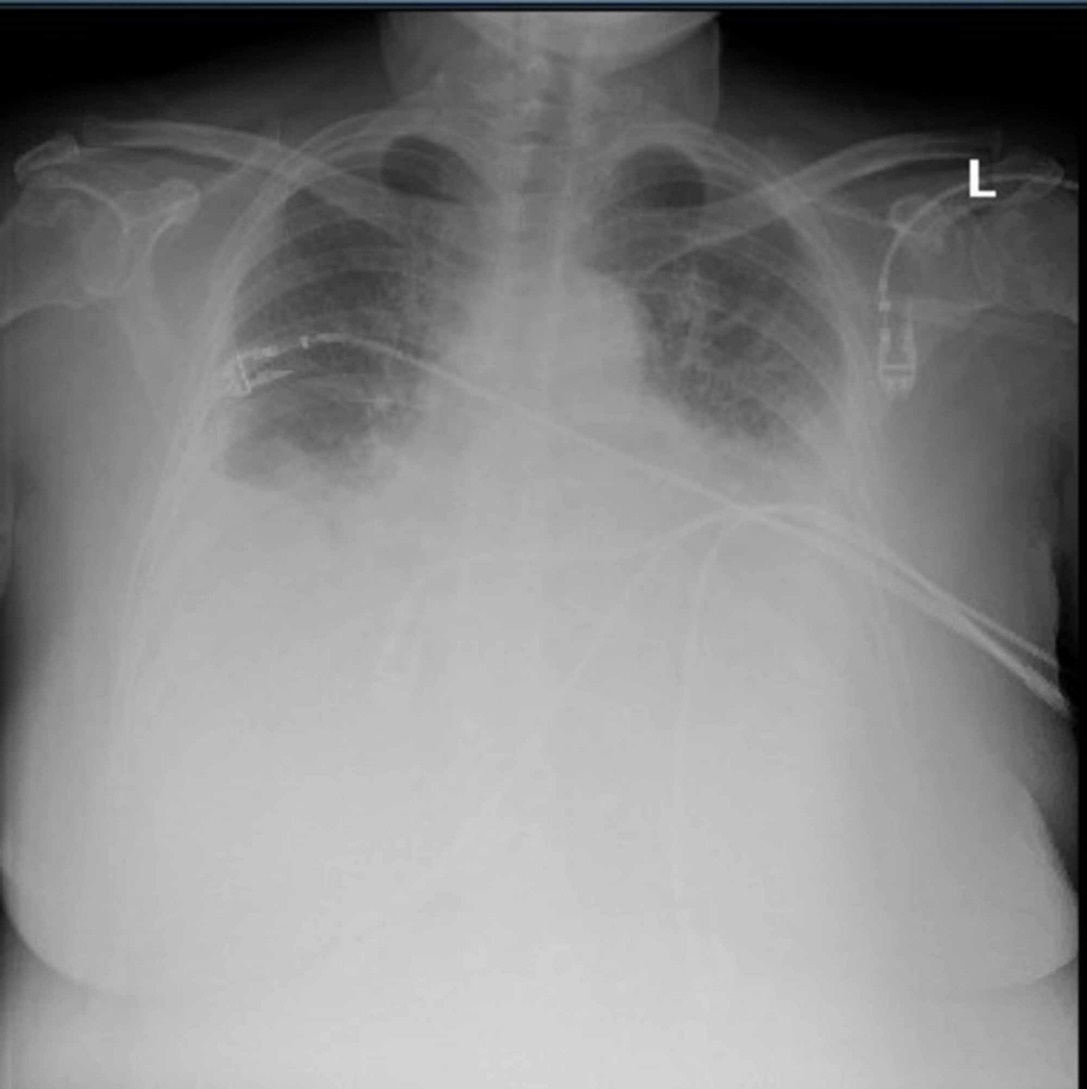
Chest xray showing small to moderate bilateral pleural effusions with associated basal consolidation/atelectasis and mild pulmonary vascular congestion suggestive of congestive heart failure.
